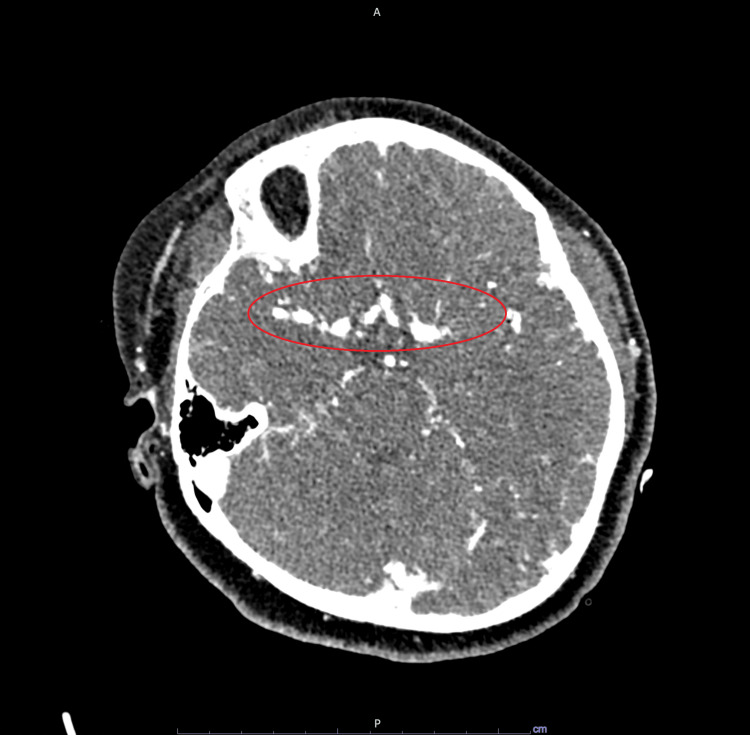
Contrast-enhanced CT angiogram showing irregular appearances of the left and right proximal MCA branches (red circle), initially concerning for vasculitis versus RCVS CT: computed tomography, MCA: middle cerebral artery, RCVS: reversible cerebral vasoconstriction syndrome
